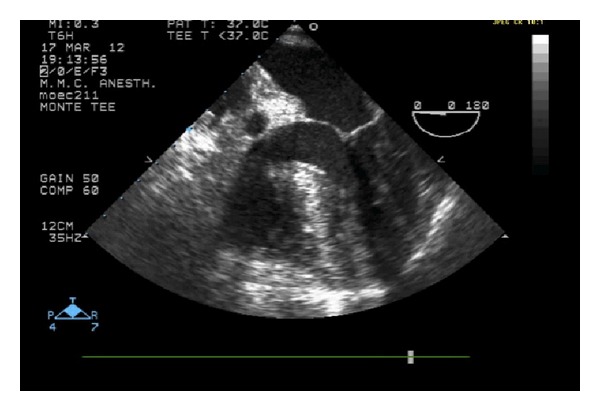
Transesophageal echocardiogram showing thrombus in the left ventricle.
